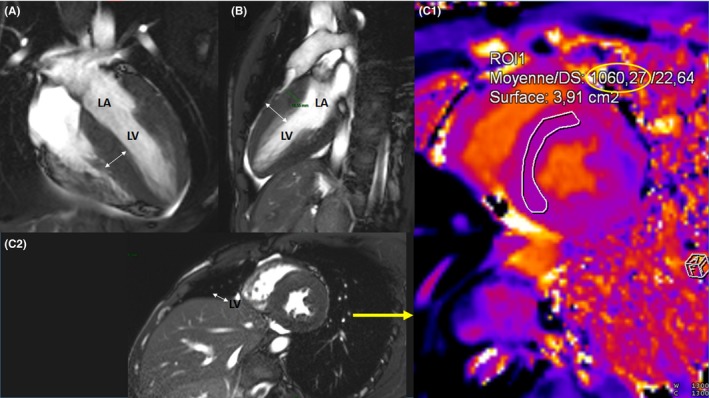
MRI acquisition at baseline showing prolonged T1 mapping consistent with the diagnosis of amyloidosis. (A) and (B) Apical 4‐ and 2‐chamber views by MRI; (C1) Short axis view by MRI; (C2) T1 mapping = 1060 ms (Normal <900 ms); LA, left atrium; LV, left ventricle
